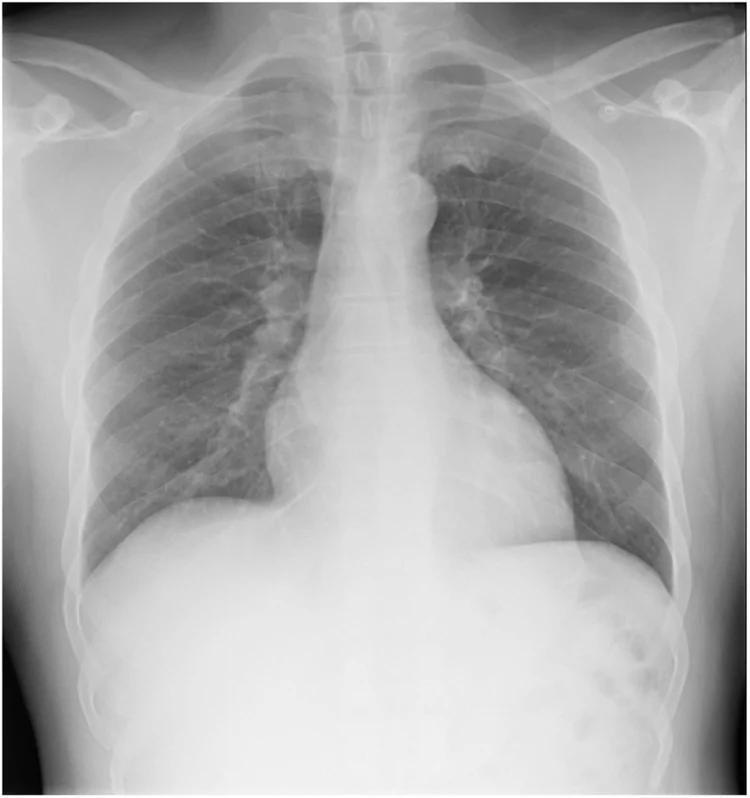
X-ray of healthy chest. Cite mimic cxr V2.1.0.
